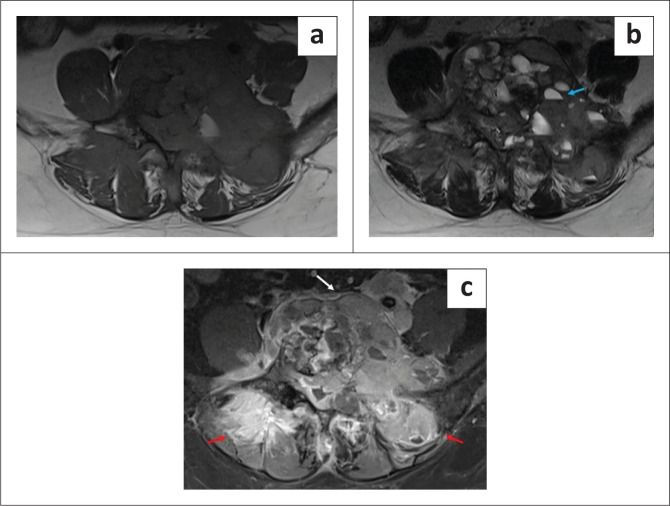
MRI axial T1-weighted (T1WI), T2-weighted (T2WI) and post-contrast T1 Fat Saturated sequence (T1FS). (a–c) demonstrate an expansile lesion of altered signal intensity involving the body of the L5 vertebra, appearing hypointense on T1WI and T2WI with marked post-contrast enhancement. There is involvement of the left transverse process, left lamina and superior articular processes of the L5 vertebra. (b) The lesion shows multiple fluid-fluid levels with a supernatant hyperintense component and a dependent hypointense component suggestive of haemorrhage (blue arrow). (c) An associated large enhancing soft tissue component is seen involving the prevertebral and paravertebral regions and bilateral erector spinae muscles (red arrow). Anteriorly, the mass is partially encasing the common iliac vessels (white arrow).
